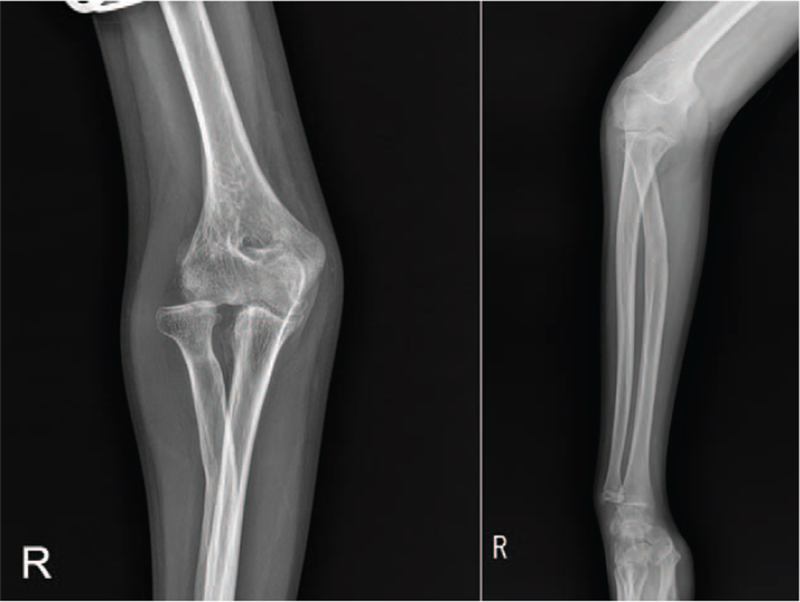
Radiographs performed at 6 wks after injury. The anterior convex deformity of the radial shaft increased to 16°, although radial head dislocation was not apparent radiographically.
